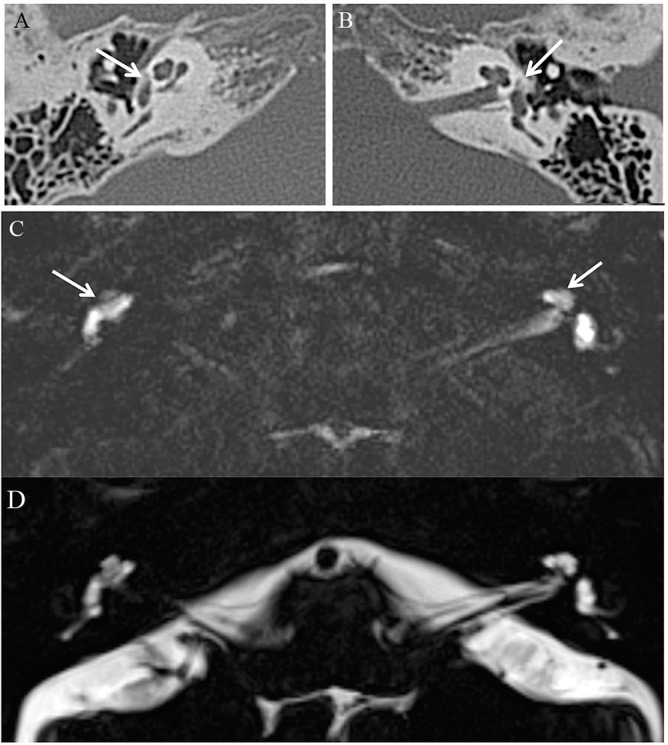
A 27-year-old woman with bilateral fenestral otosclerosis. (A, B) On computed tomography images, otospongiotic plaques anterior to the oval window are seen as small low density areas (arrows). (C) Heavily T_2_-weighted three-dimensional fluid-attenuated inversion recovery (3D-FLAIR) image obtained 4 hours after intravenous administration of a single dose of gadolinium-based contrast agent (IV-SD-GBCA). Remarkably high signal is seen bilaterally in the cochleae (arrows). In this particular subject the signal intensity ratio (SIR) was 27 in the right cochlea and 39 in the left cochlea. (D) Magnetic resonance cisternography (MRC) at the slice level corresponding to that in (C).
